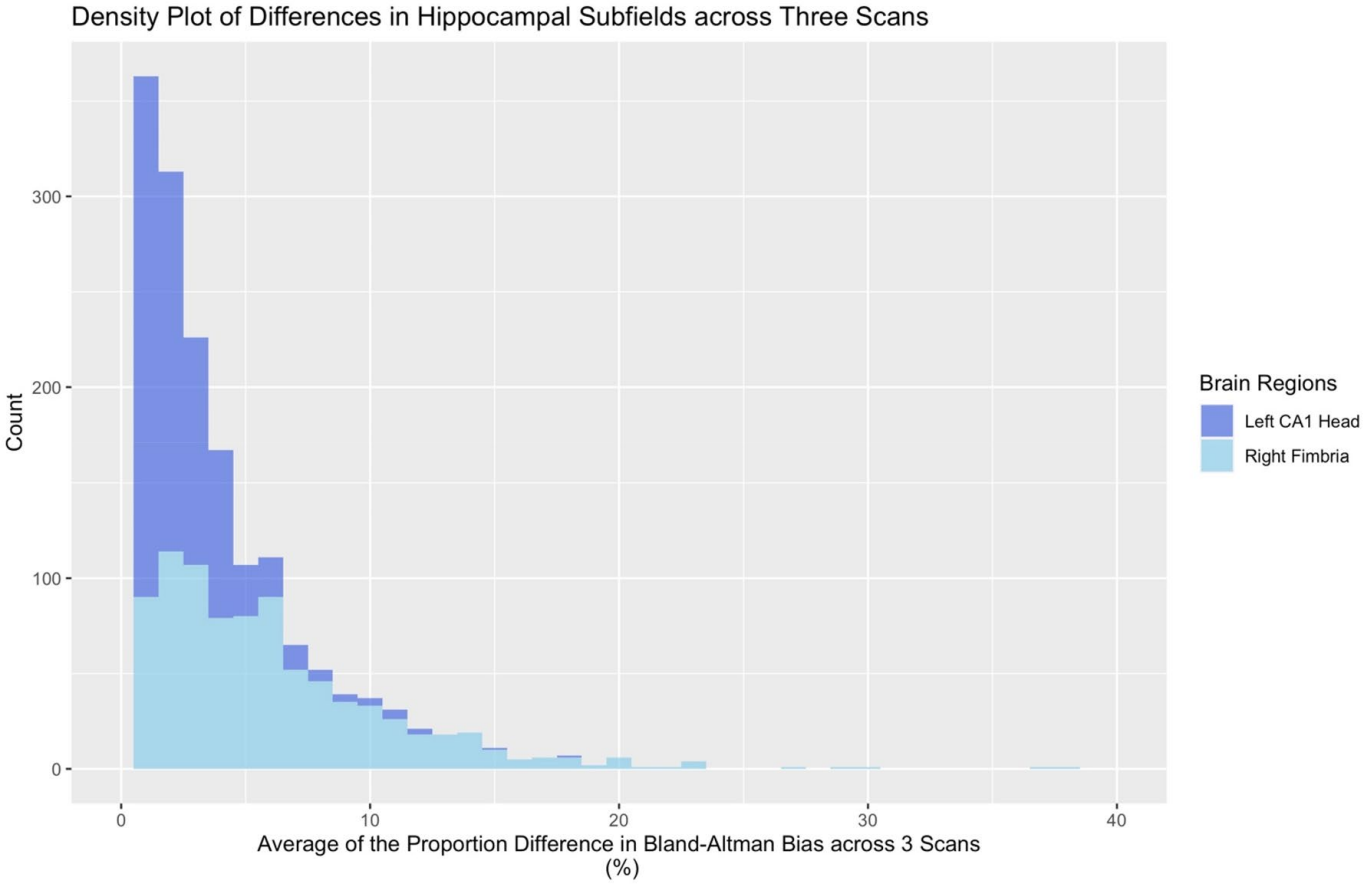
Bland–Altman plots of the average difference for volume estimation across subjects’ three MRI scans for the Left Cornu Ammonis (CA) 1 Head (dark blue) and Right Fimbria (light blue). The horizontal axis indicates the average difference in Bland–Altman “*bias*” (difference between subregional volume output for different scans, as a proportion of a region’s volume), while the vertical axis indicates the number of scans with a given value. Of note, the left CA1 Head has a low degree of mean bias (as a proportion of the region’s volumes; 0.106%), while the right Fimbria has a fair degree of mean bias (1.198%)
